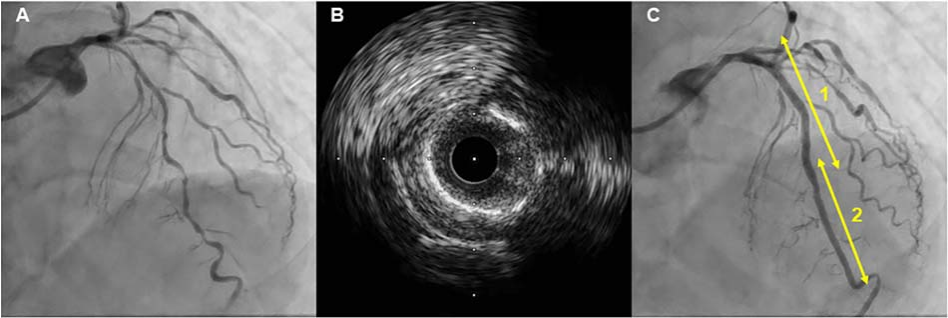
First PCI: Angiogram of LAD showed diffuse severe stenosis (A) and calcified plaque on intravascular ultrasound (B), and two stents (1; XIENCE Skypoint 3.0 × 48 mm, 2; XIENCE Skypoint 2.25 × 15 mm) were implanted (C).
